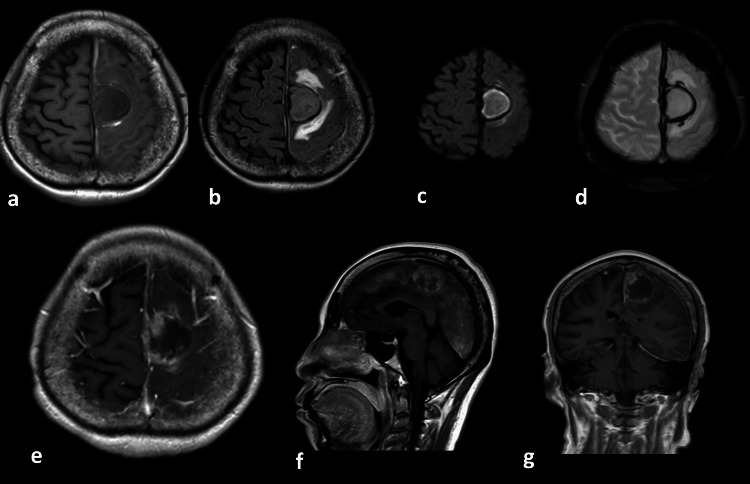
MRI images after hemorrhage a: T1 weighted image; b: FLAIR; c: DWI; d: T2-star weighted image; e-g: Gadolinium-enhanced MRI (e: axial image, f: sagittal image, g: coronal image) The images show brain parenchymal edema and hemorrhage around the tumor (b). A previously absent high signal on DWI was visible within the tumor (c). Enhancement was observed along the cerebral falx but not in most of the tumor's interior (e-g). FLAIR: fluid-attenuated inversion recovery; DWI: diffusion-weighted image
